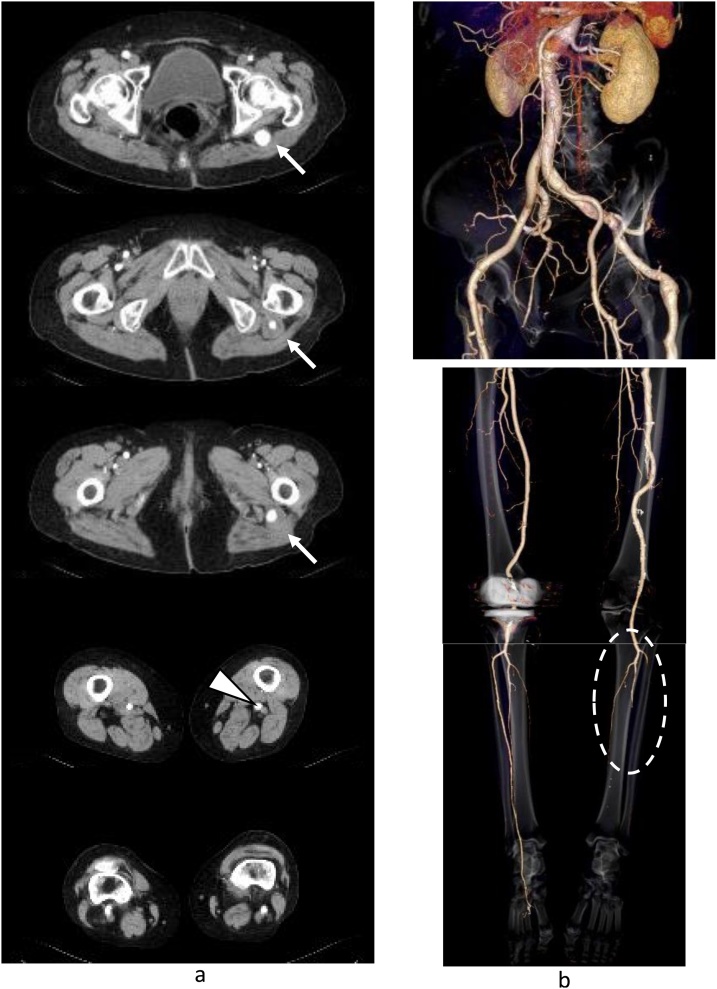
CT images at first admission. a. CT shows a left persistent sciatic artery (PSA) aneurysm (arrow) and the left popliteal artery located more laterally than the normal pattern (arrow head). b. Three-dimensional CT shows complete-type PSA, hypoplastic superficial femoral artery (7 mm), and occlusion in the three artery branches of the lower leg (circle).
